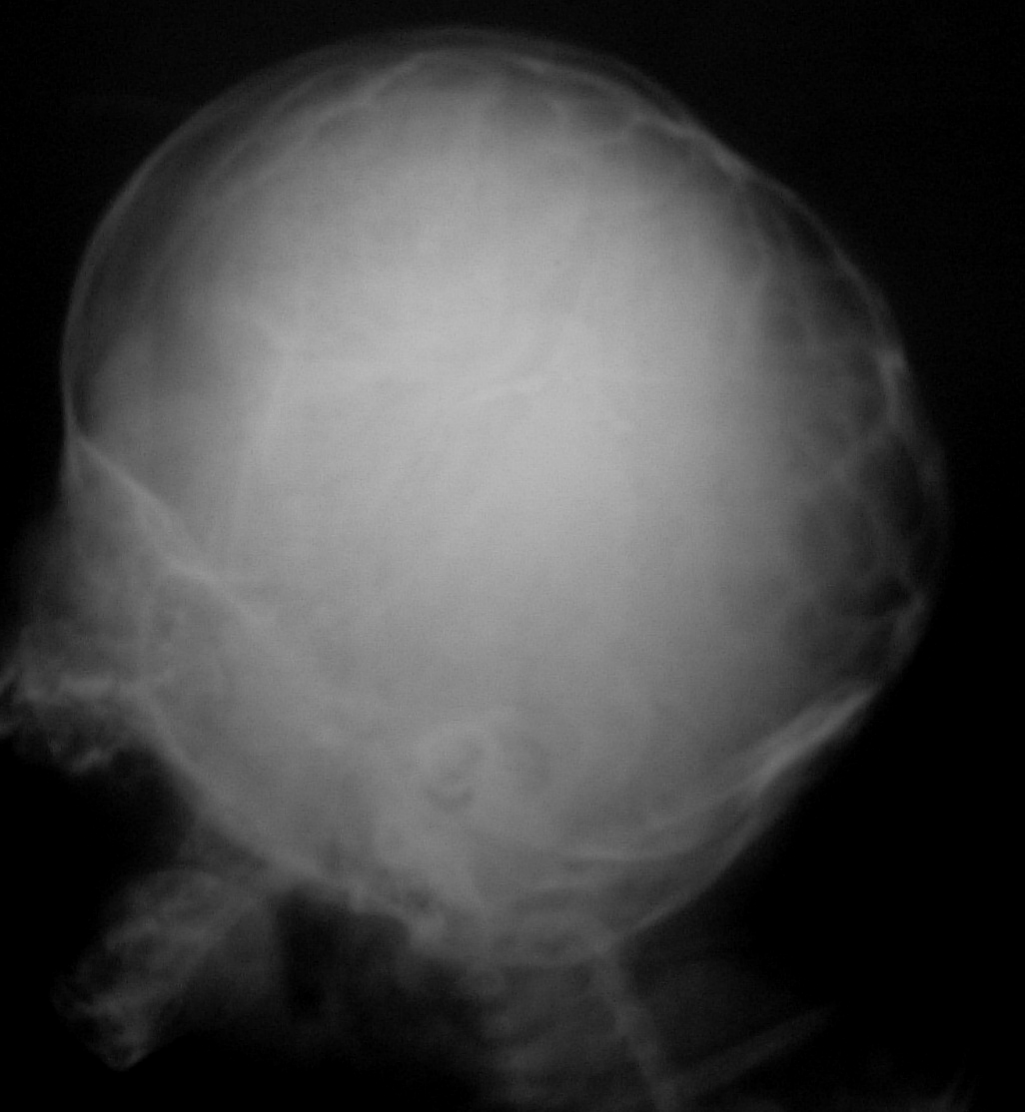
Skull X-ray shows fusion of both coronal and lambdoid sutures with diffuse beaten copper appearance.
